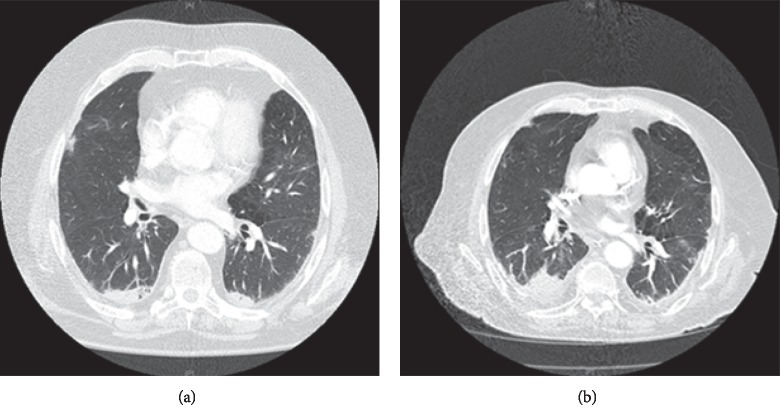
(a) CT pulmonary angiogram of patient 2 reveals bilateral calcified pleural plaques, subpleural fibrosis, paraseptal emphysematous change, and a small region of honey combing in the right lower lobe base laterally. (b) CT chest six months later revealing interval increase in the size of the consolidation, resolution of the right upper lobe spiculated lesions, and stable pulmonary fibrosis.
